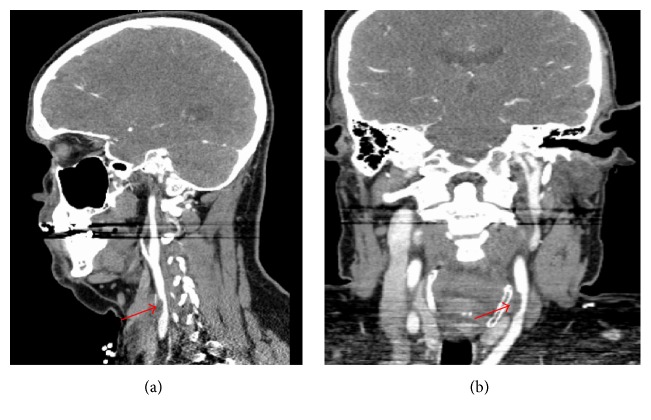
Sagittal (a) and coronal (b) CT angiogram of the head and neck demonstrating a 1.5 × 0.8 cm focal adherent mural thrombus (red arrows) in the left common carotid artery 4 cm proximal to the bifurcation resulting in less than 50% stenosis of the luminal diameter.
